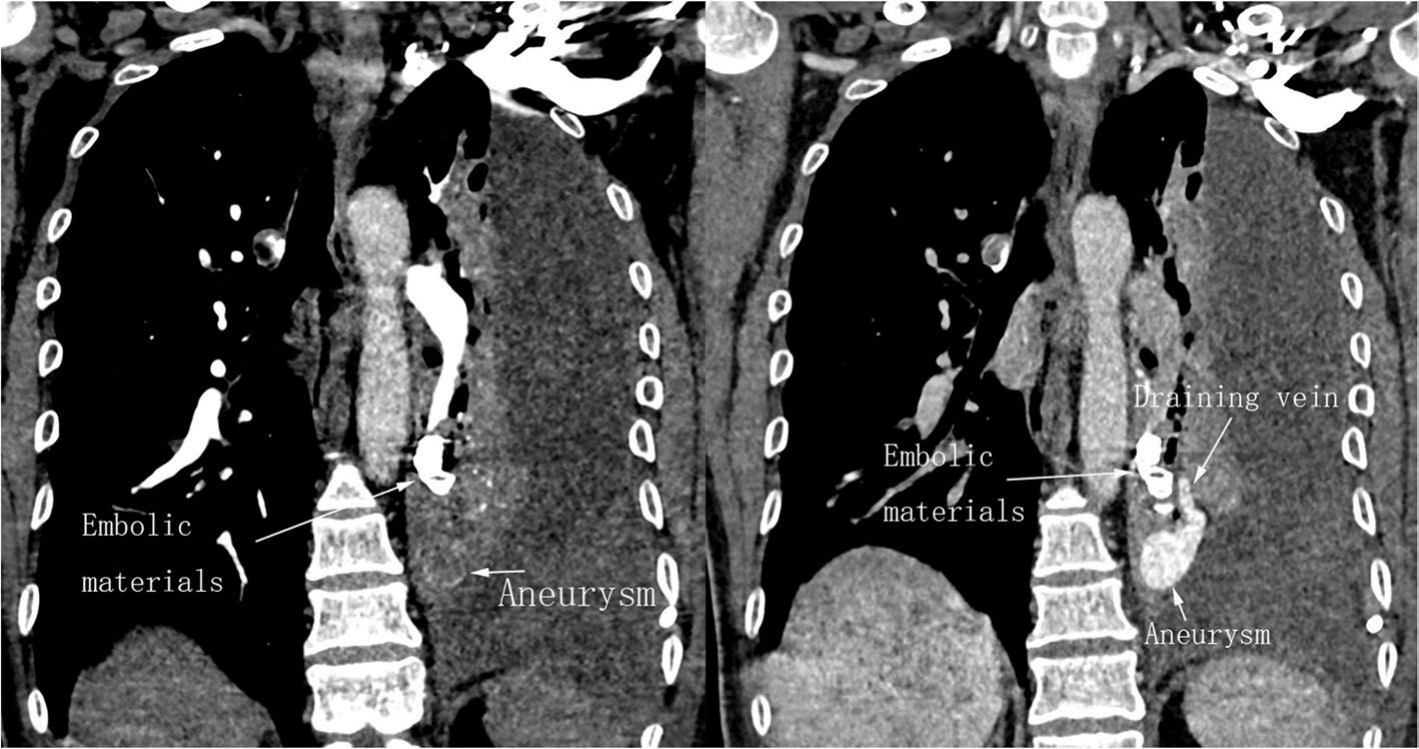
A-B Follow-up contrast-enhanced CT showed a large amount of fluid accumulation in the left pleural cavity, partial lung collapse and remnant of arteriovenous fistula in the delayed phase
